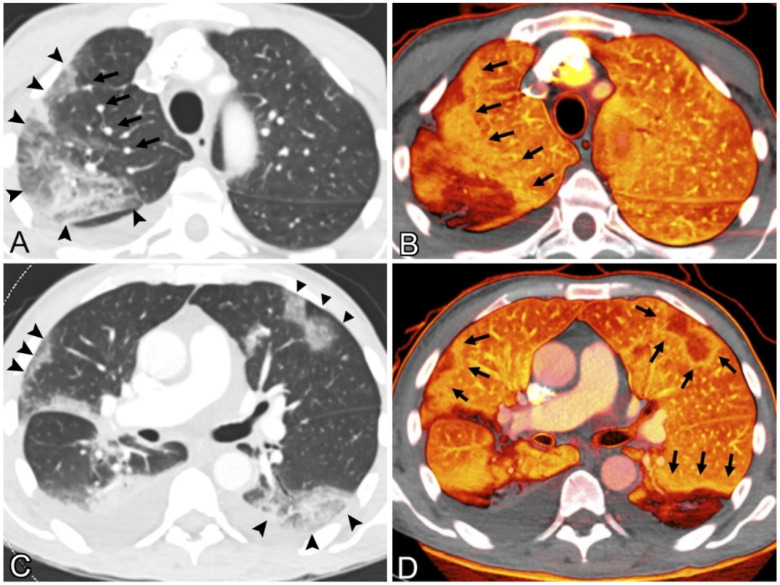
(A) 69-year-old man with fever, weakness, and chills had coronavirus illness. The patient was hospitalized for acute intermittent tachycardia, desaturation, and shortness of breath. No pulmonary emboli were found. Contrast-enhanced CT pulmonary angiography of the upper lungs at lung windows showed ground-glass opacity and consolidation in the right upper lobe (arrowheads); sub-segmental arteries within the opacities were dilated, and right upper lobe vessels proximal to the opacity were similarly dilated (arrows). (B) Pulmonary blood volume (PBV) imaging at the same level shows a significant peripheral perfusion deficiency with a surrounding halo of enhanced perfusion (arrows). Heterogeneous left upper lobe perfusion. CT scan of the patient’s lower lungs showed peripheral ground-glass opacities and consolidation with a round or wedge-shaped appearance (arrowheads). (D) PBV picture shows perfusion deficiencies matching the opacities in (C), shown with enlarged perfusion halos (arrows) [82] (2020).
